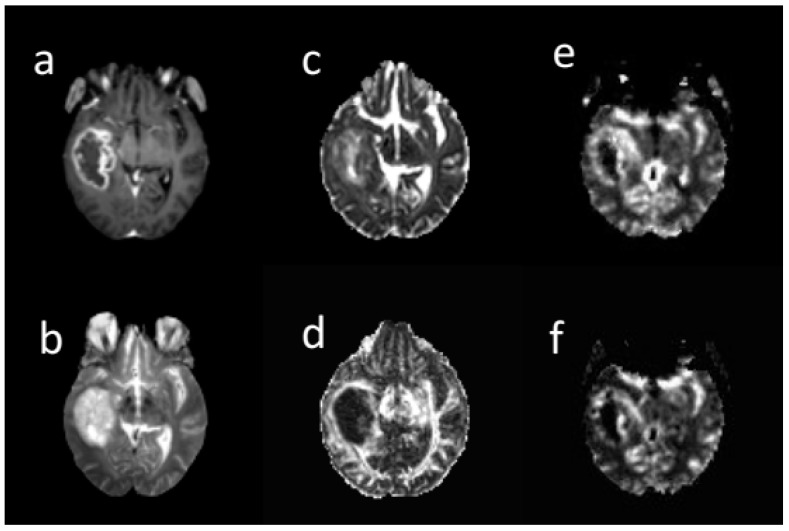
A sample of different brain MRI images: (a) post-contrast T1-weighted, (b) T2-weighte, (c) MD map, (d) FA map, (e) CBV map, (f) CBF map in 66-years-old woman with glioblastoma. All images are co-registered to the B0 DTI images. The tumor region was segmented into contrast enhanced, non-enhanced, necrosis in post-contrast image, low and high diffusion in MD map, and low and high perfusion in rCBV map by k-means. Based on their overlapping, sub-regions were obtained.
